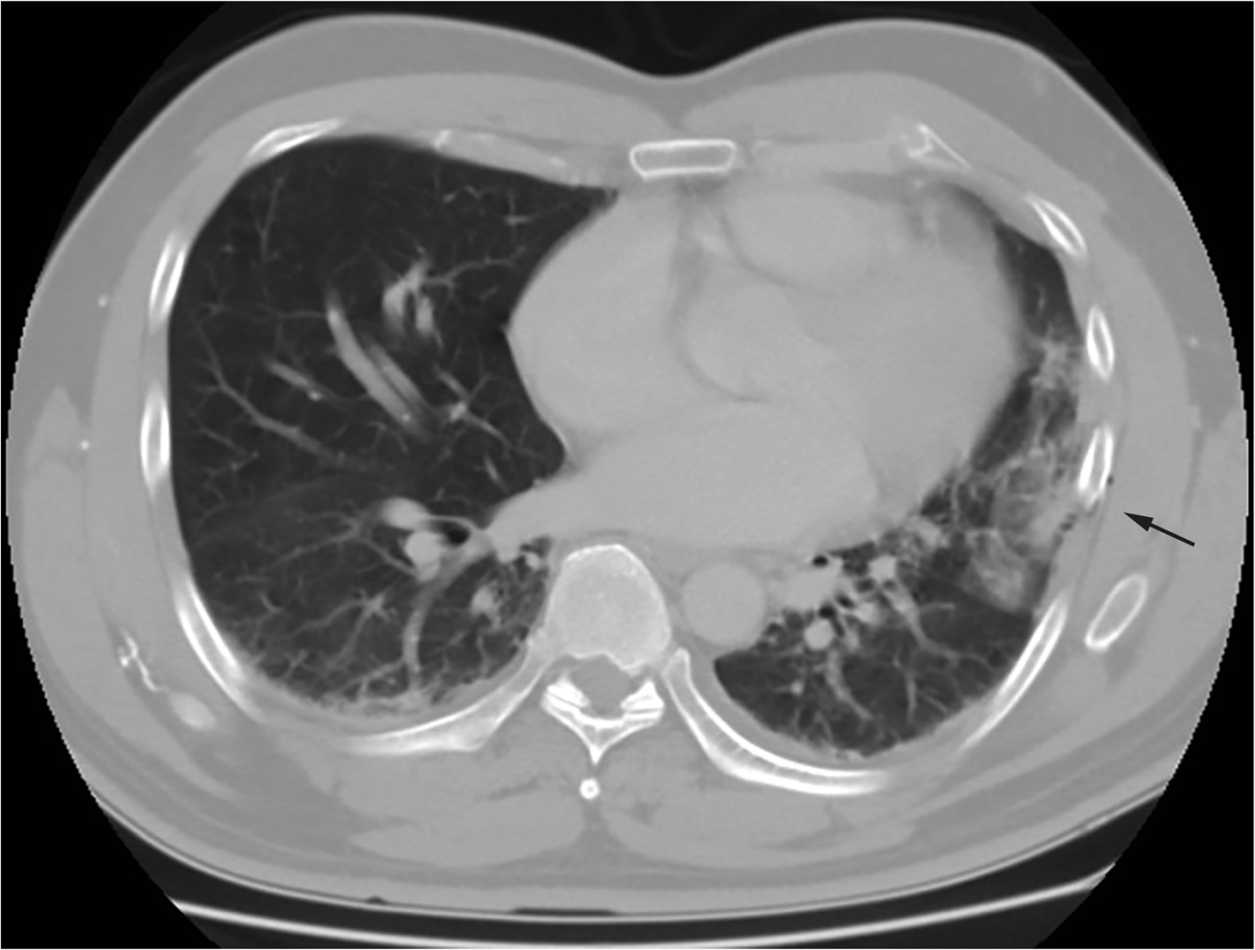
Computed tomography (CT) scan of the chest reveals lung atelectasis with intercostal extension of the lung parenchyma (arrow) secondary to being trapped by the left 6th rib
